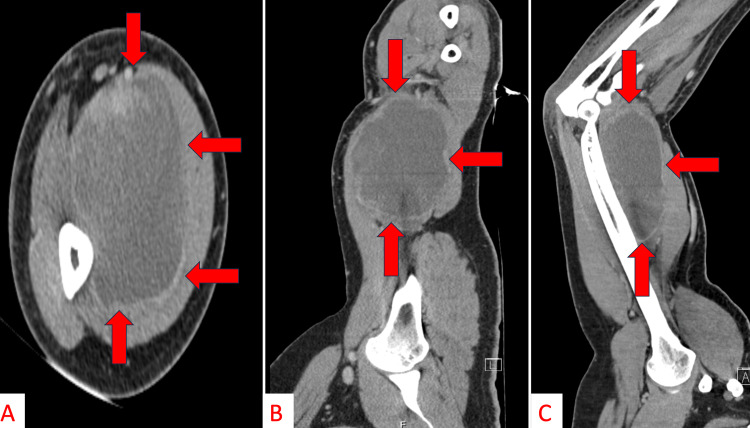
Axial (A), coronal (B), and sagittal (C) CT-scan images with contrast displaying a mildly irregularly marginated/enhancing heterogeneous lesion measuring up to 15 cm overlying the distal anteromedial humerus (red arrows indicate borders of the pseudocapsule). The radiologist reported, “the primary differential considerations included evolving hematoma and Morel Lavallee lesion given the recent history of trauma, cannot exclude an evolving abscess."
